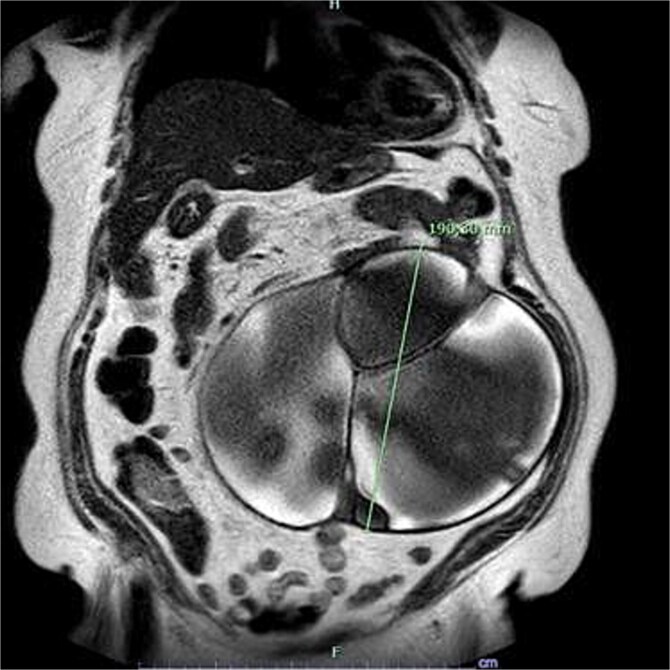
The view of magnetic resonance imaging of the mass (a multiloculated complex cystic mass occupying a large portion of the abdominal cavity and extending inferiorly to the pelvic inlet).
